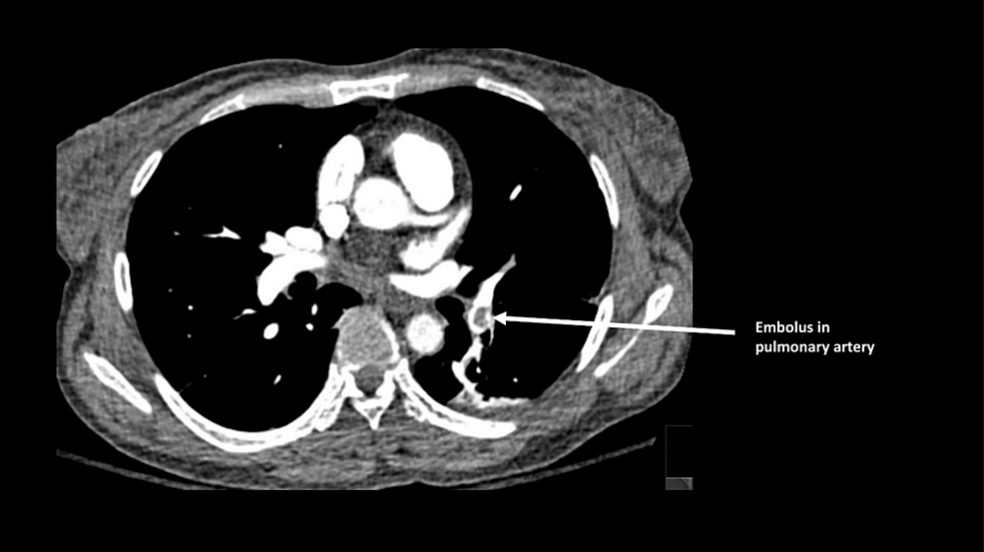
Axial view of computerized tomographic (CT) pulmonary angiogram showing a radiolucent clot in the left pulmonary artery depicted by the arrow
